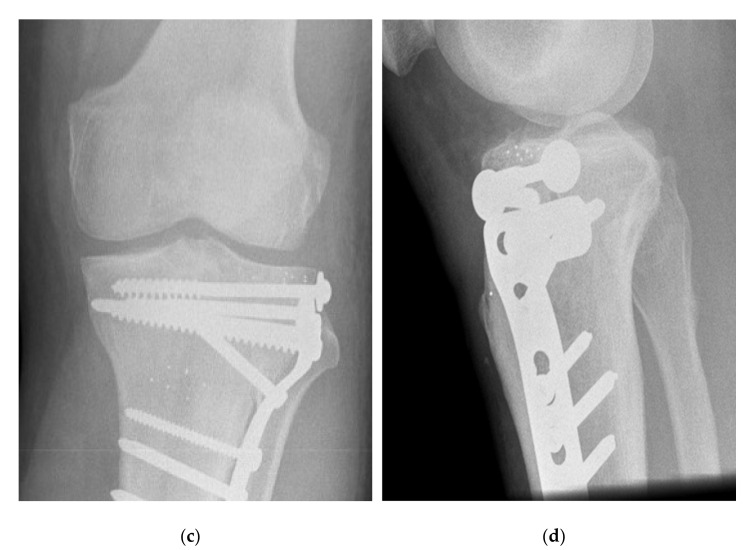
Preoperative AP (a) and lateral (b) with postoperative Day 2 AP (c) and lateral (d) radiographs of a reduced tibial plateau fracture (Schatzker II) in a patient without medical comorbidities.
